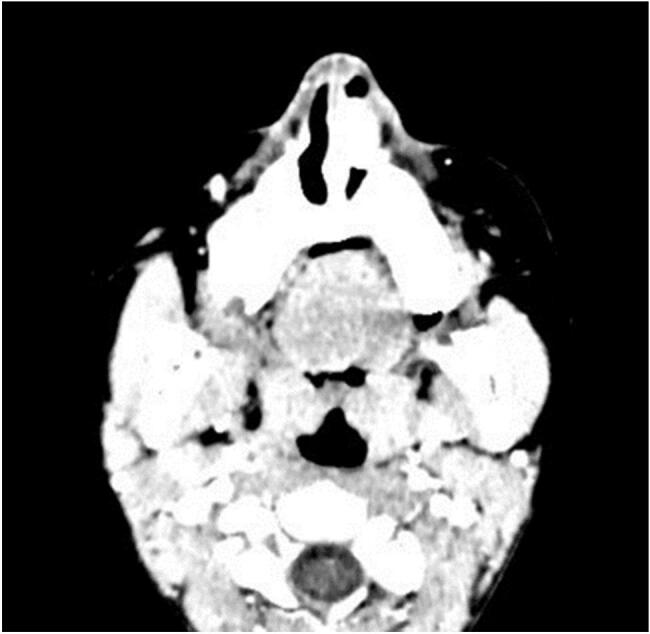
CT nose and paranasal sinuses showed rounded soft tissue hyperdense lesion arising from the left side of floor of the anterior portion of the nose with intense post contrast enhancement with density of 275 HU (Axial Cut).
